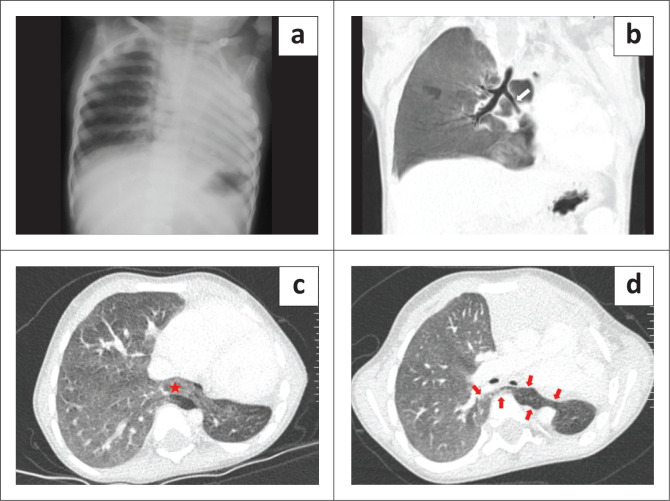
Horseshoe lung in a 2-year-old male associated with left lung hypoplasia. (a) Chest radiograph demonstrates a small, opaque left hemithorax with ipsilateral mediastinal shift and compensatory hyperinflation of the right lung. (b) Minimum intensity projection (MinIp) coronal image of the CT chest shows a hypoplastic left main stem bronchus (white arrow) with an absent left upper lobe branch. (c and d) Axial CT chest (lung window) demonstrates fusion of both lungs (red star) without any intervening fissure, with the isthmus of the midline lung parenchymal tissue (red arrows) extending up to the level of the tracheal bifurcation.
